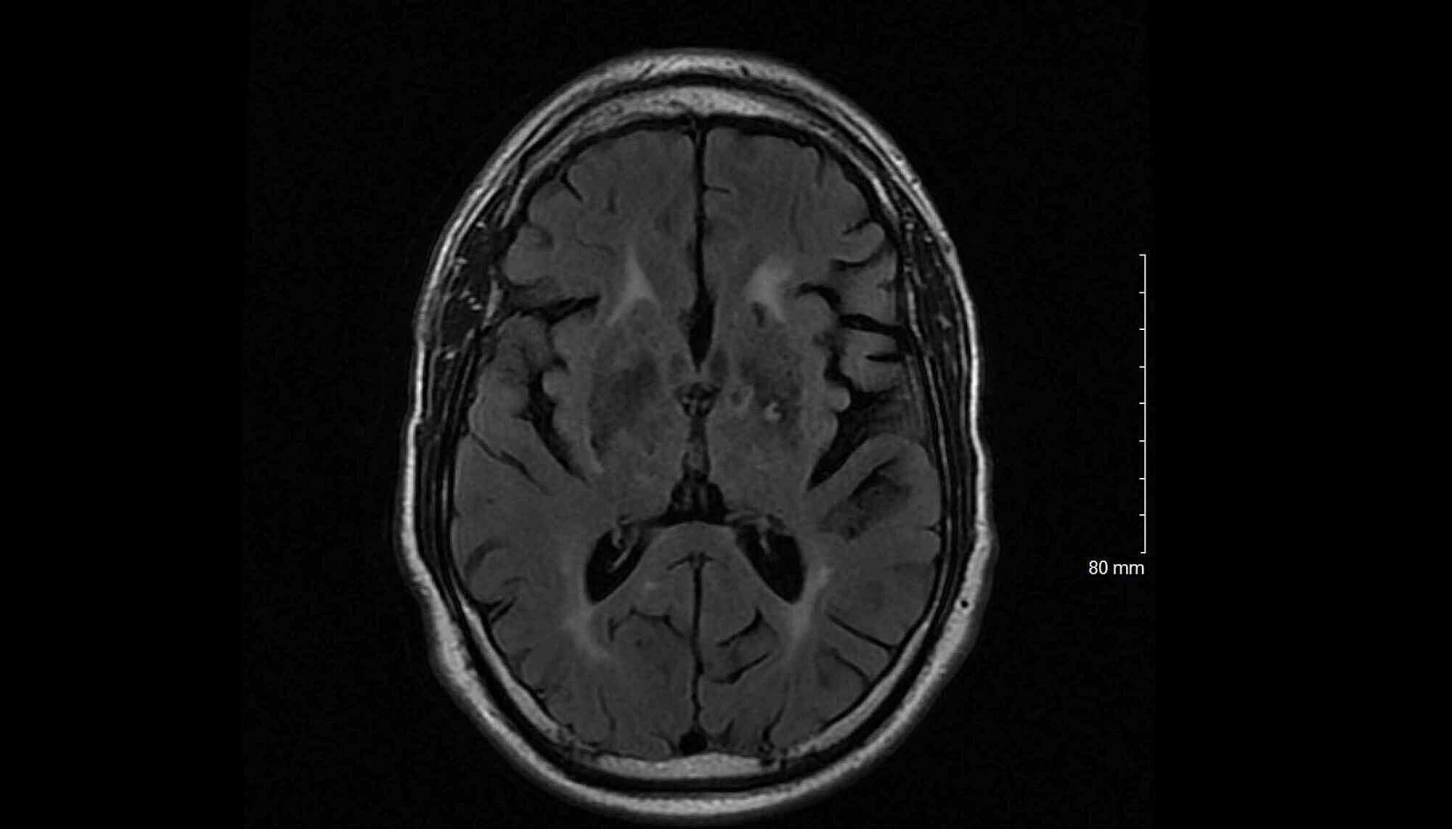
Non-contrast MRI brain shows hypointensity in the posterior limb of the left internal capsule consistent with an infarct and bilateral white matter hyperintensities consistent with microvascular changes in axial T2 FLAIR imaging. FLAIR: fluid-attenuated inversion recovery.
